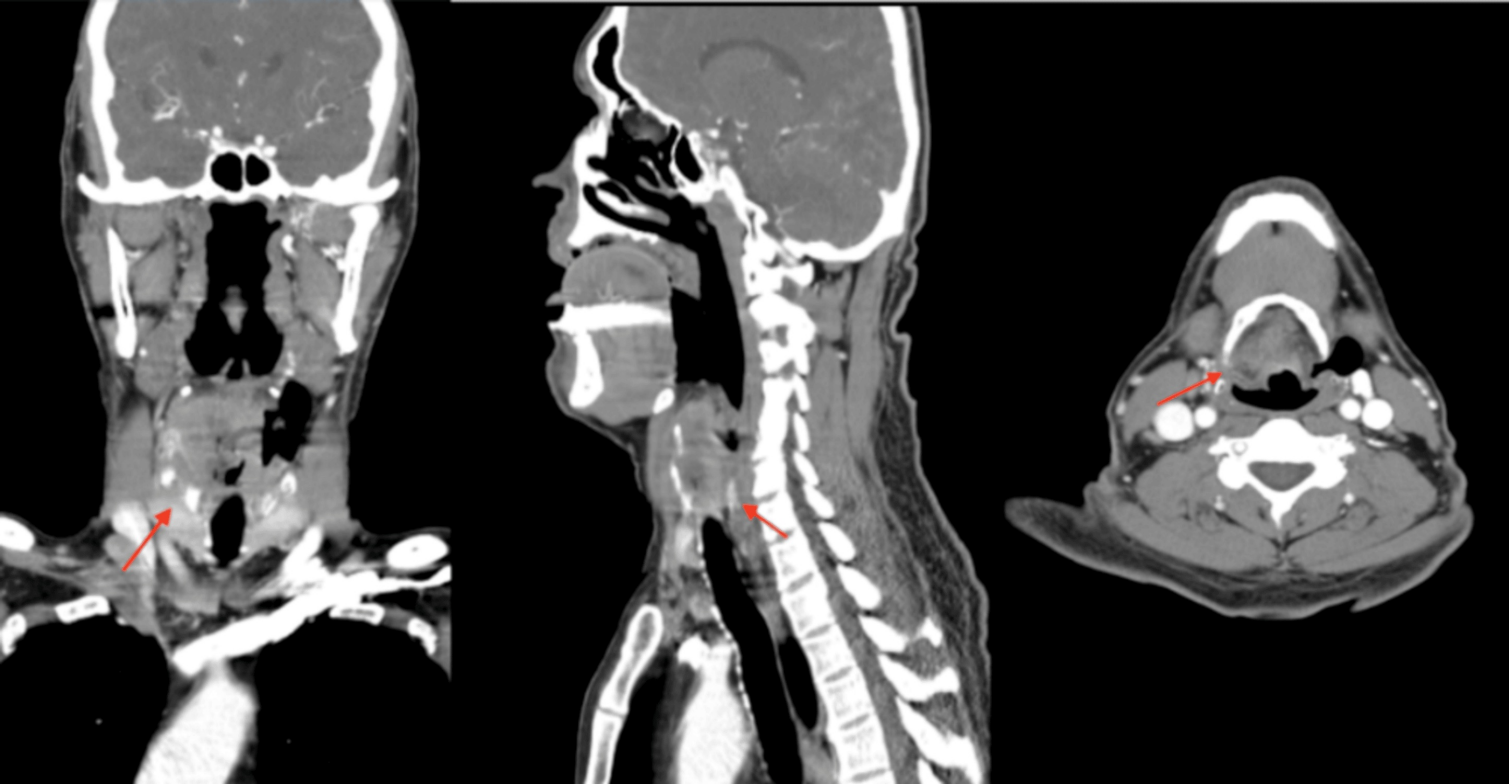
CT scan of the head and neck (multiple views) A CT scan of the head and neck shows supraglottic mass extending to the vocal cords and appears to be extending around the lateral aspect of the true vocal cords, with some narrowing of the vocal cord level (red lines).
